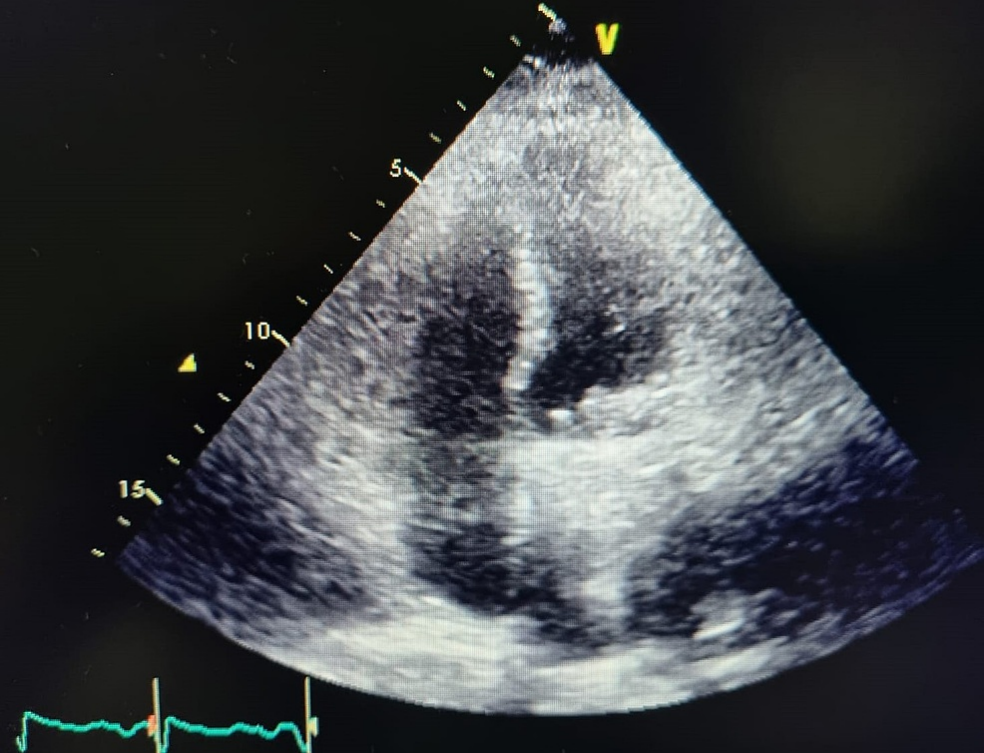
Color Doppler echocardiogram A hyperechogenic (with acoustic shadowing), mobile image of 7 x 9 mm is shown at the lateral level of the mitral prosthesis toward the ventricular side, one of the discs showed excursion without excursion of the second disc.
